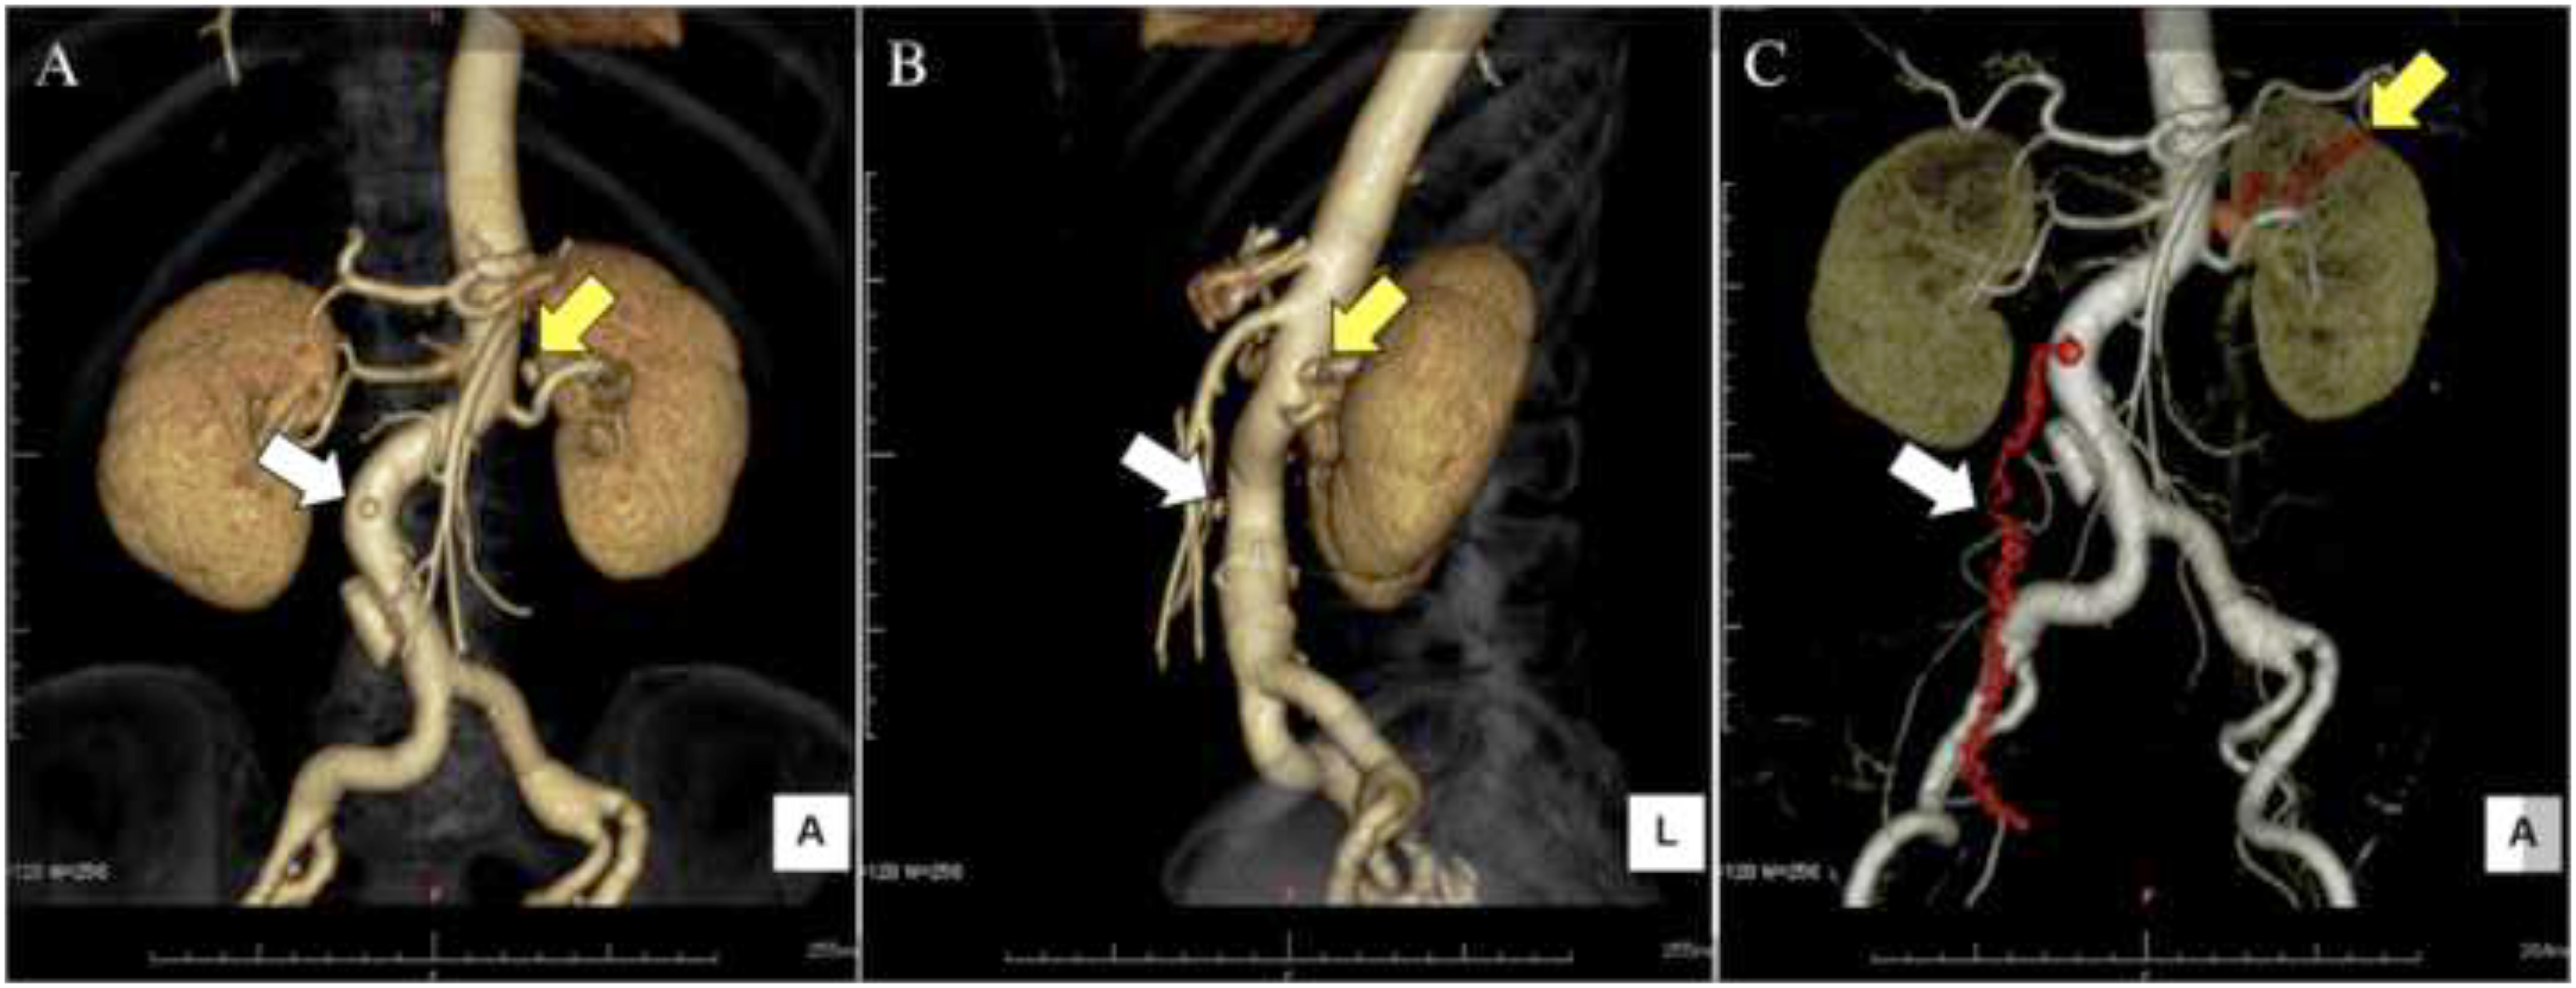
Fig. 2 Three-dimensional computed tomography (A, C: frontal view; B: lateral view) on day 11 show collateral retrograde flow from the aortic branches considered as the left middle suprarenal (yellow arrows) and right gonadal arteries (white arrows).
